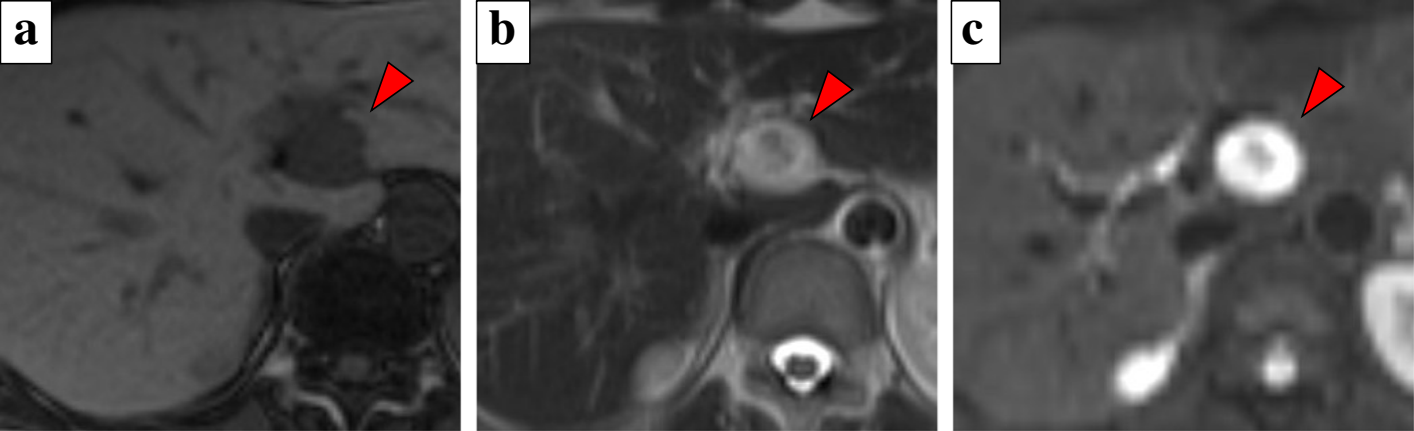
Magnetic resonance images showing a tumor with lower intensity compared with the liver on T1-weighted images (a, *red arrowhead*), and with higher intensity than the liver on T2-weighted and diffusion-weighted images (b and c, *red arrowhead*)
